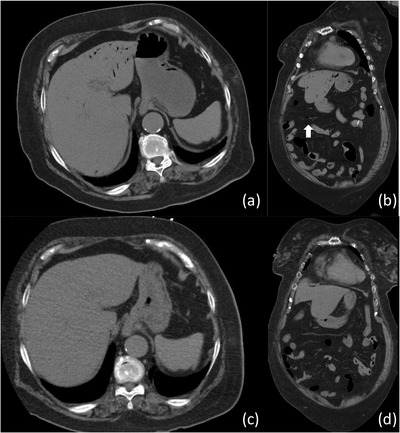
(a, b) Computed tomography revealed the presence of hepatic portal venous gas (HPVG) and gas in the middle colic vein (arrow) without pneumoperitoneum. (c, d) a follow‐up computed tomography showed disappearance of HPVG and gas in the middle colic vein
